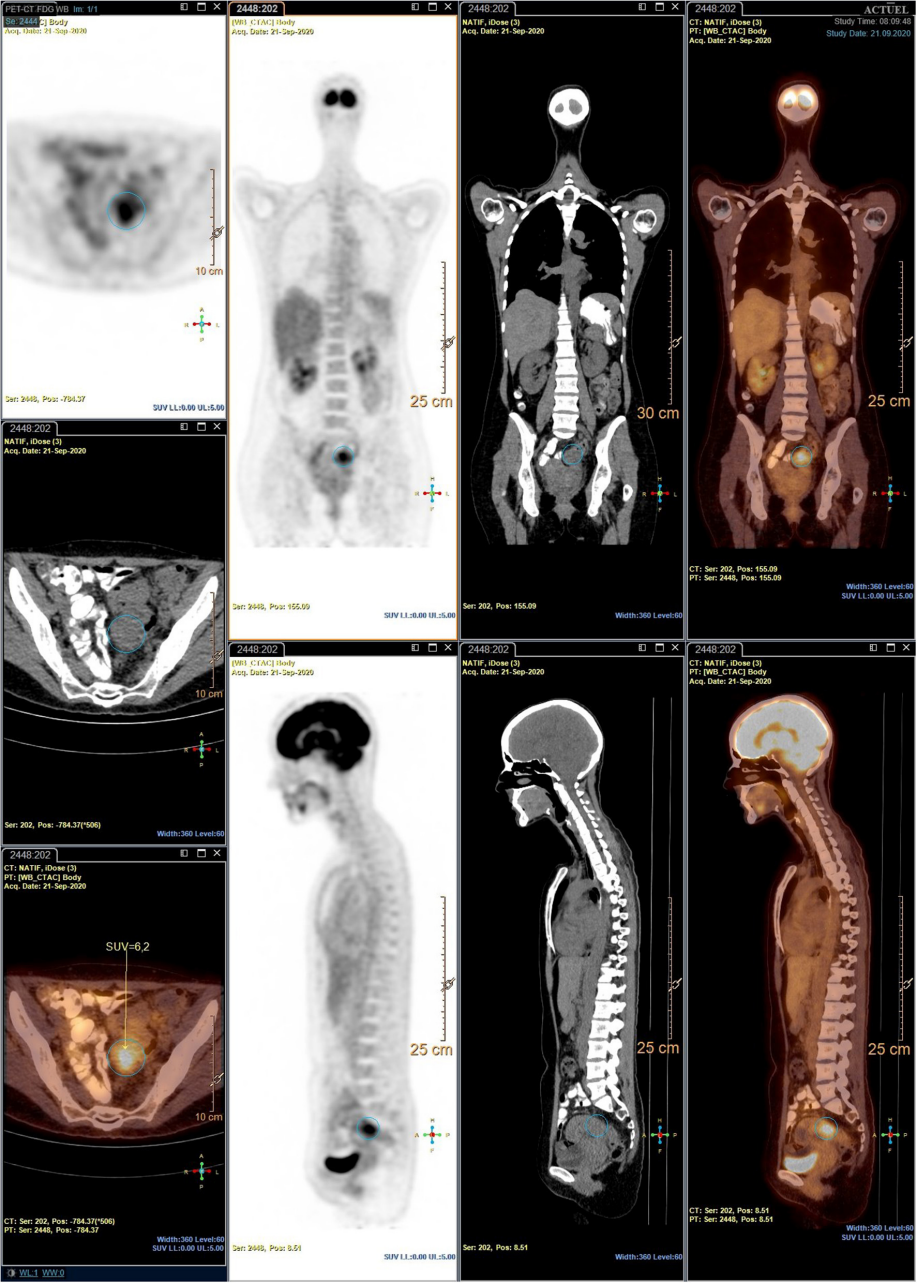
18F-FDG PET-CT, pre-treatment with Bevacizumab: intense recto-sigmoid hypercaptation (circled in blue) without further hypermetabolic lesions.
